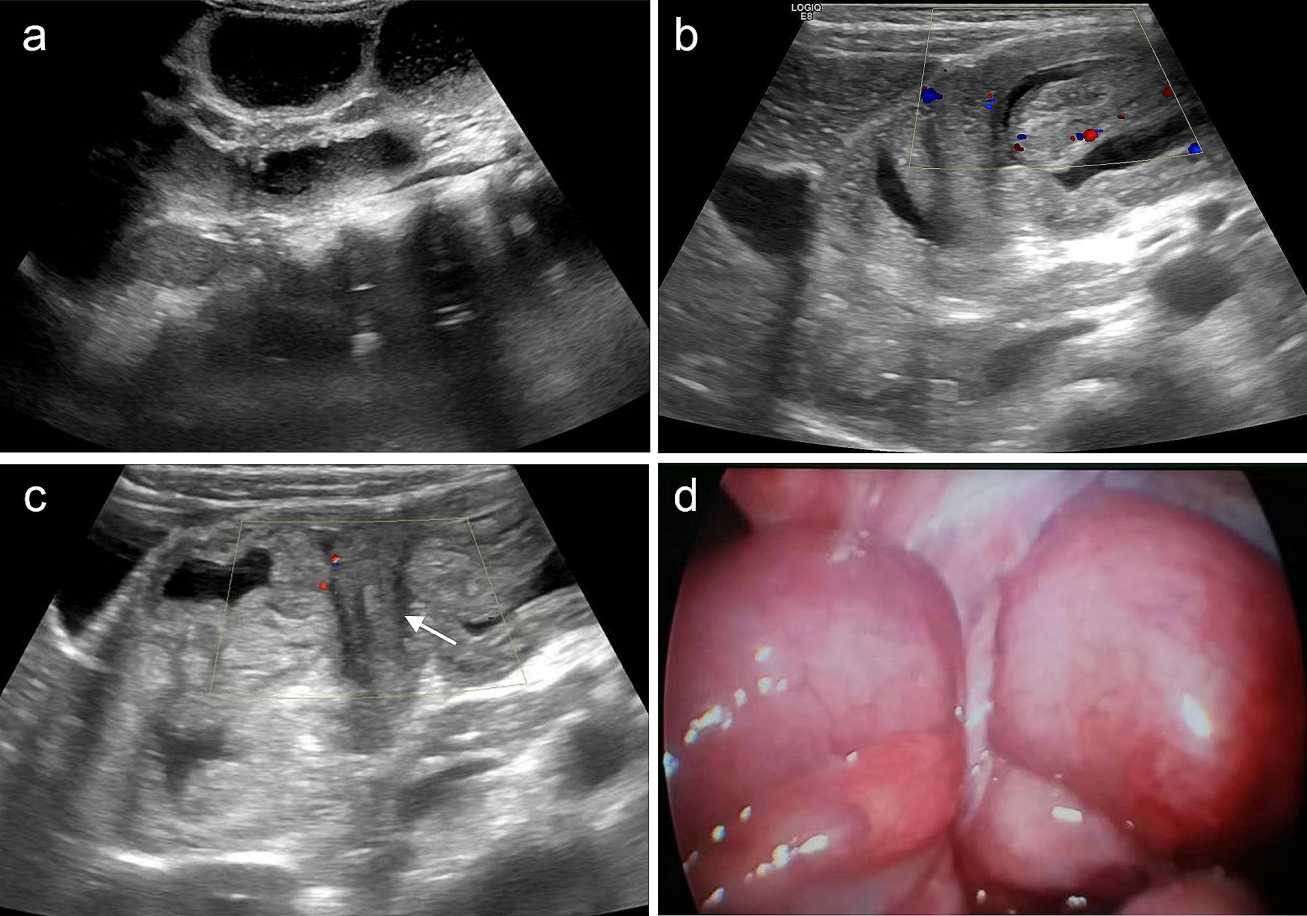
Representative imaging scans of a 5-year-old female patient with an IAH. A: Fluid accumulation in the small bowel loops visualized on the ultrasound scan, considering the intestinal obstruction. B: Ultrasound scan showing significant edema and thickening of the intestinal wall, and CDFI showing a reduced blood supply. Postoperative diagnosis of an IAH caused by adhesive band and secondary intestinal obstruction was made. C: Postoperative appendicitis, adhesive bands and the herniated bowel were visualized. The wide low echo (white arrow), short adhesive bands and dilation of the intestinal tubes at both ends constitute a typical “cross sign”. D: Postoperative appendicitis, adhesive banding with the greater omentum (white arrow) and dilation of the herniated intestine at both ends were shown
